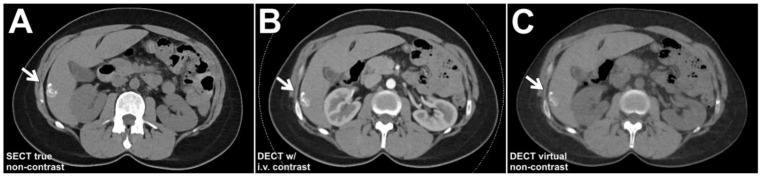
A 42-year-old woman with a history of metastasized breast cancer. A calcified liver lesion (arrows) was visible in the true non-contrast phase of the single-energy baseline CT (SECT; (A)). The dual-energy follow-up CT scan with intravenous contrast enhancement (DECT; (B)) allows for calculation of virtual non-contrast images that can replace the true non-contrast scan in depicting the sclerotic hemangioma in liver segment 6 (C).
